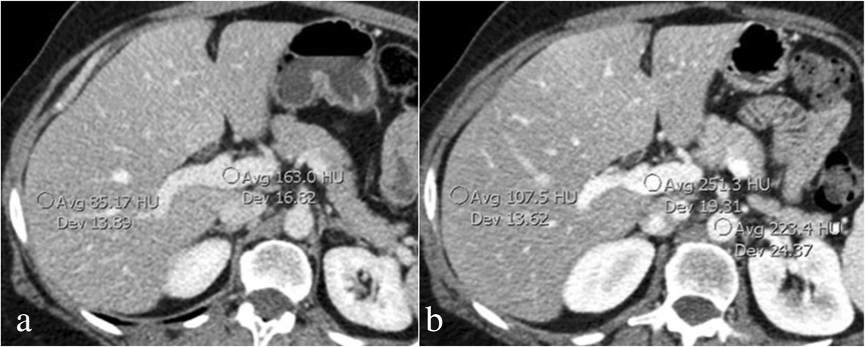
Circular region of interest measurements of portal vein and liver parenchyma performed during portal venous phase (a) of the triphasic computed tomography and during combined phase (b) of the single-pass split-bolus computed tomography. Mixed phase (hepatic arterial phase/portal venous phase during hepatic enhancement) of the split-bolus multidetector-row computed tomography shows a higher attenuation of the portal vein and liver parenchyma with respect to that of the portal venous phase of the triphasic multidetector-row computed tomography protocol. Abbreviations: Avg, average; Dev, standard deviation; HU, Hounsfield unit.
